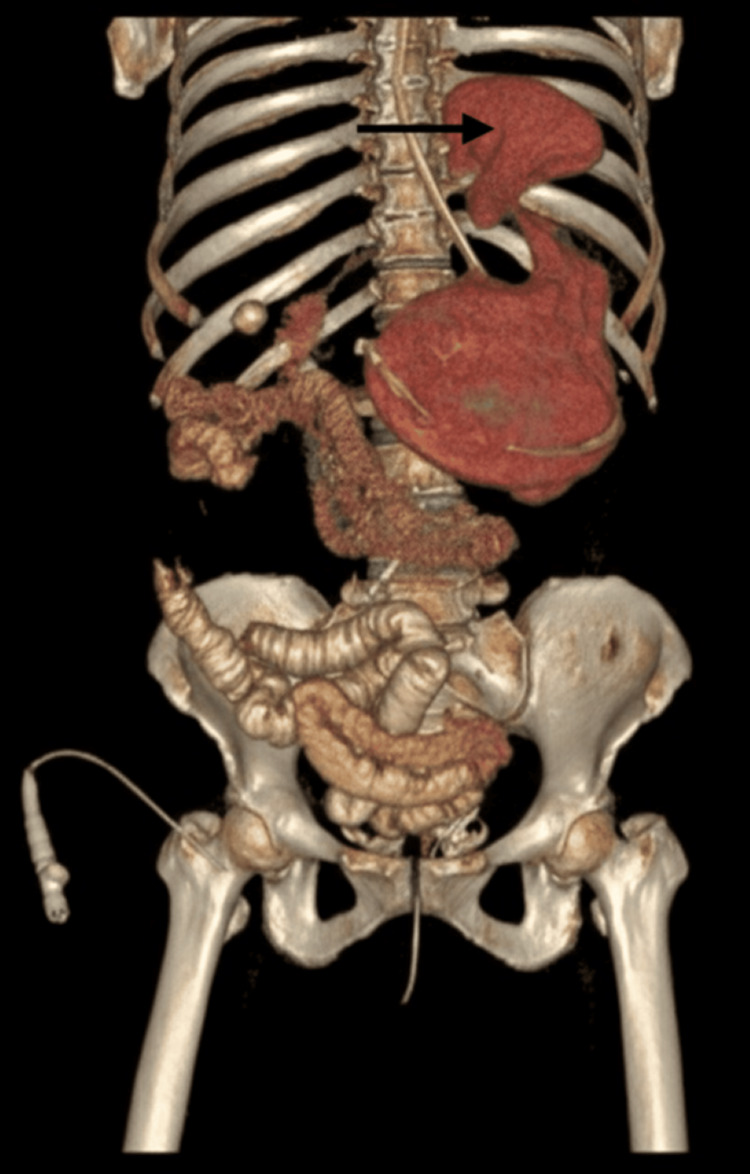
Three-dimensional reconstructed computed tomography (CT) image of the abdomen demonstrating the mesenteroaxial gastric volvulus (arrow).
